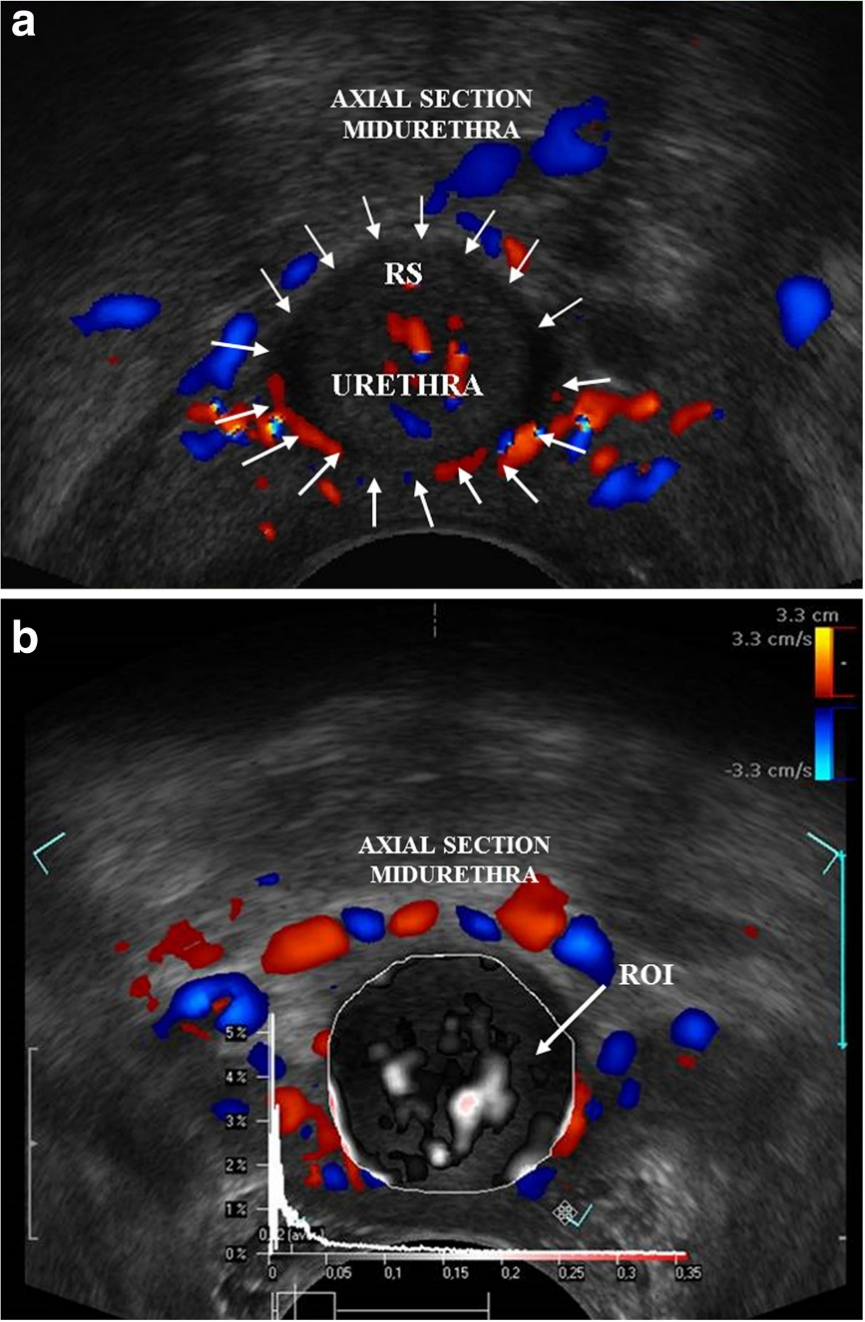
Endovaginal US and Pixel Flux technique in axial section of the mid urethra. a Endovaginal US with the use of high-frequency 180 degrees rotational transducer (type 8848, B-K Medical) in axial section of the mid urethra, colour Doppler mode. Arrows pointing the borders of the urethra. RS- rhabdosphincter. b Pixel Flux technique: Region of interest (ROI) in axial section of mid urethra showing the local perfusion relief.
